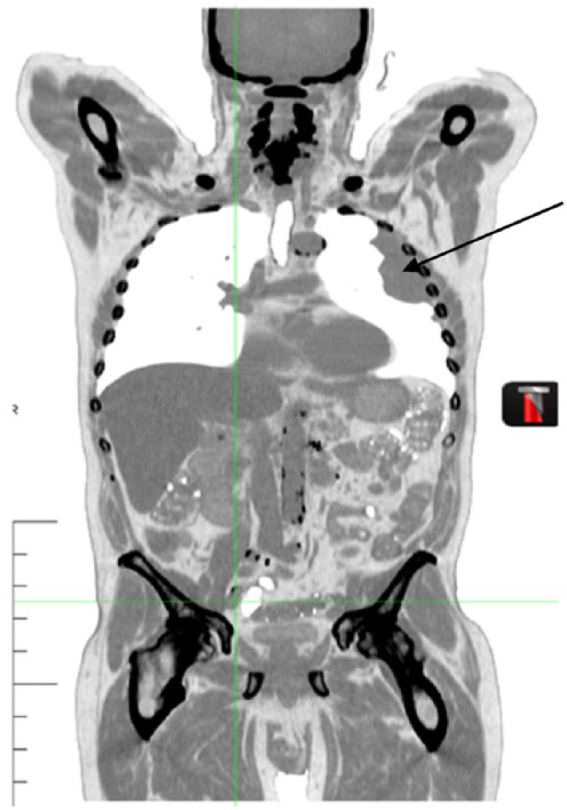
January 2011, a repeat CT scan was performed, and again the mass in the left
